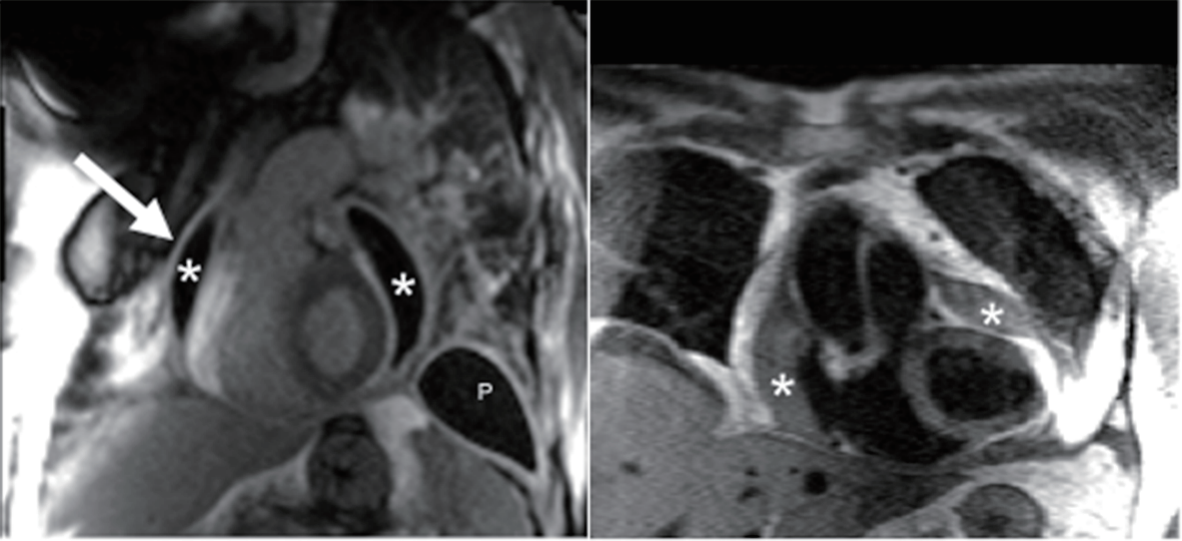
Post-gadolinium inversion recovery sequence with steady state free precession readout demonstrates a grossly thickened pericardium (arrow) accompanied by a loculated pericardial effusion (*). Pleural thickening with effusion (P) is also present. Enhancement of the pleura and pericardium is consistent with significant fibrosis. Double inversion T1-weighted turbo spin echo sequence also demonstrates the findings of a loculated pericardial effusion.
